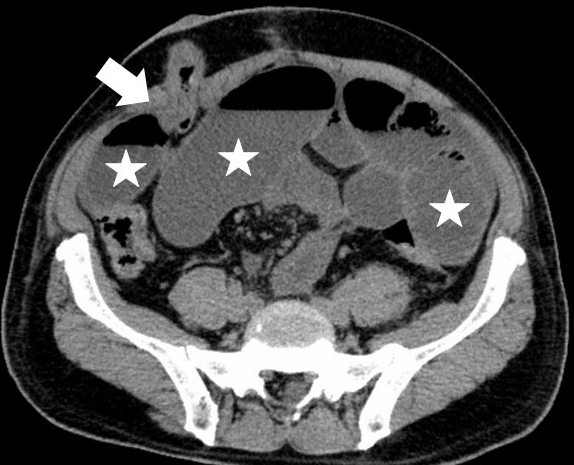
SOO patients were defined as those who had abdominal bloating symptoms and had CT findings of bowel obstruction at the stoma outlet and intestinal dilatation. White arrow head: bowel obstruction at the stoma, ☆: dilated intestines.
